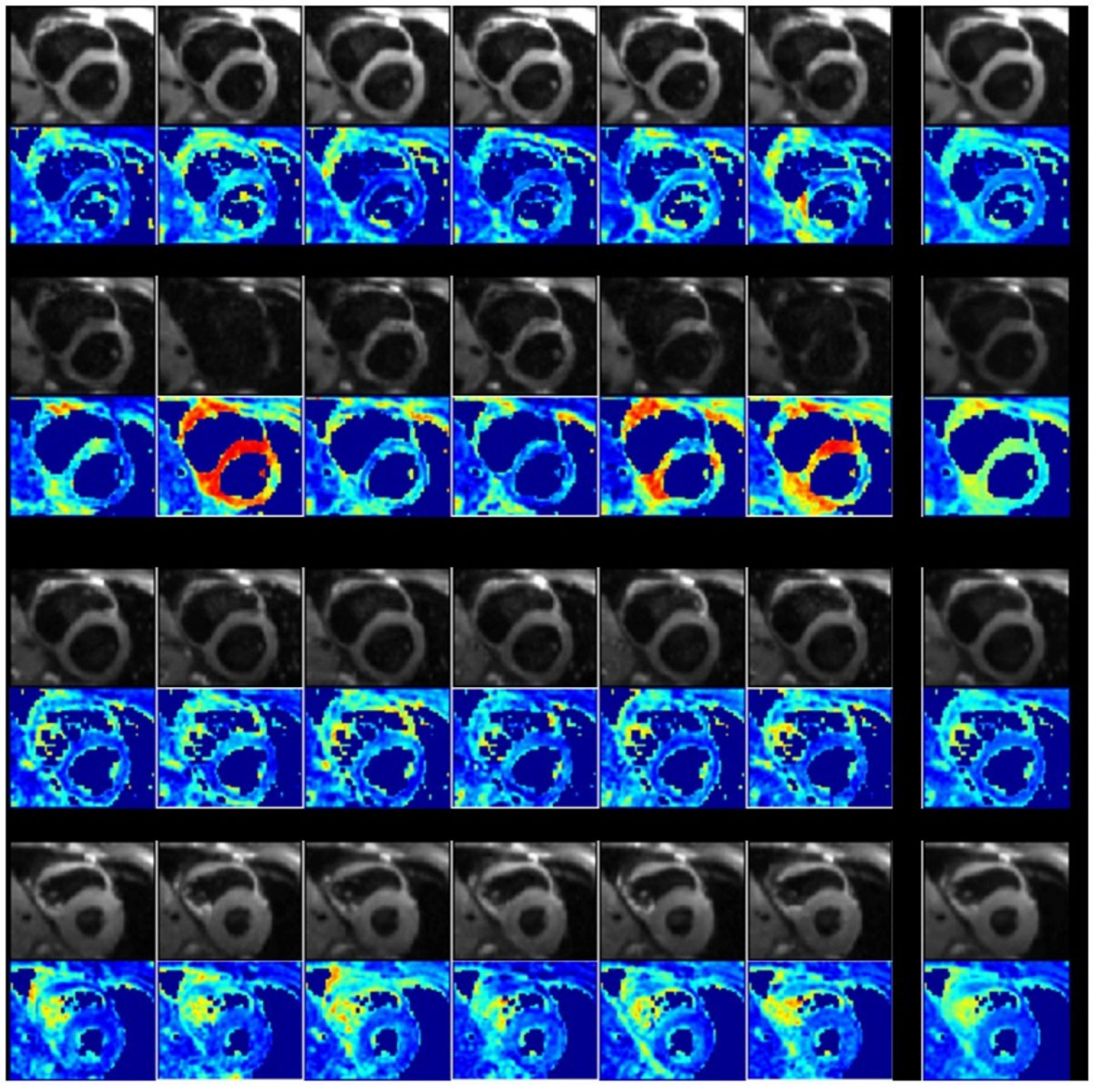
Example of ADC raw images of mid-ventricular short axis slices. In gray scale the Diffusion Weighted Image (DWI) for the 6 directions, b-value = 200 mm^2^/s and the Trace image. In color scale, the corresponding error map,corresponding to the artefact quantification ε. When the DWI signal drop out, artefact quantification reveal higher value.
